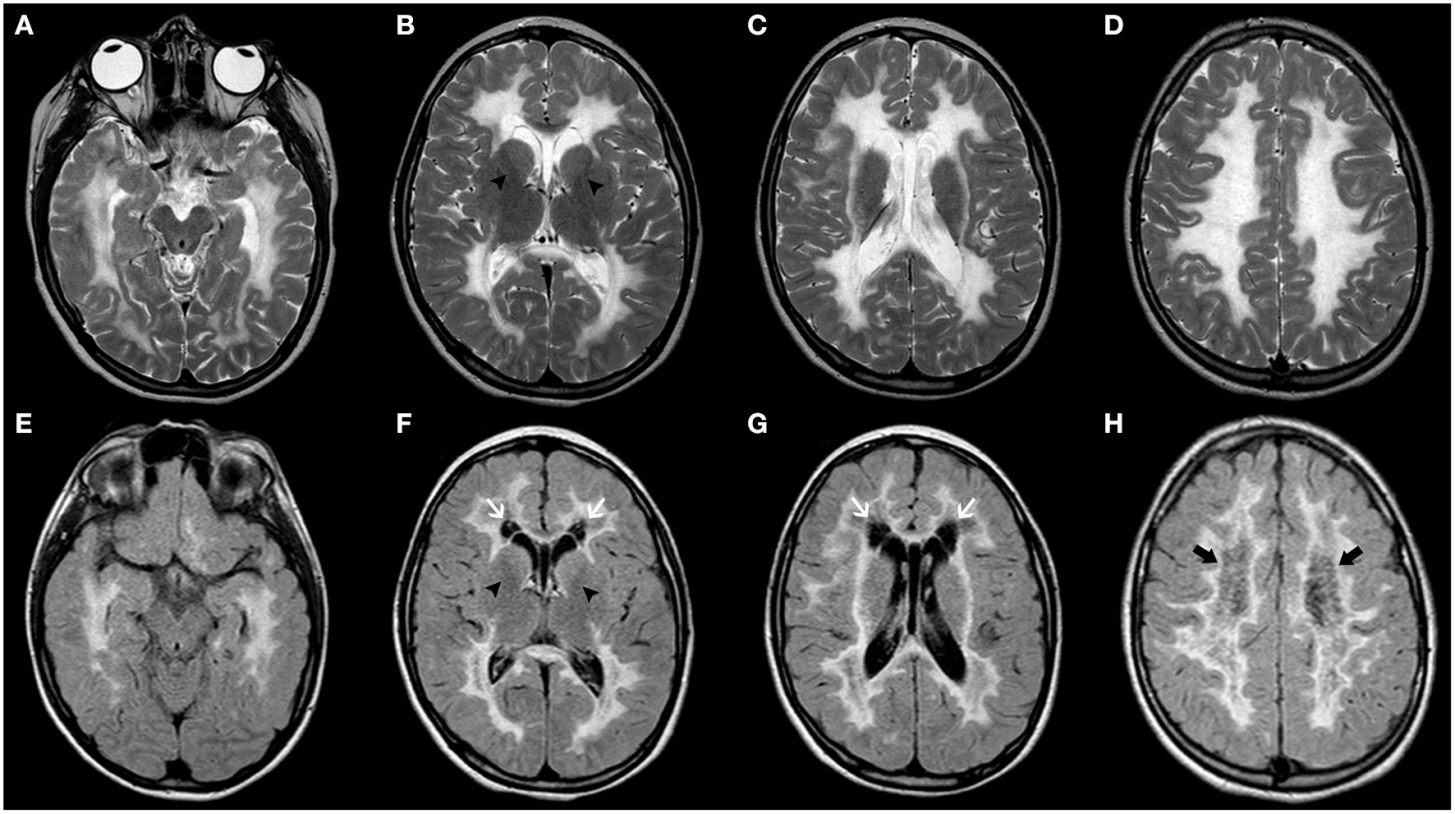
Imaging characteristics of Childhood Ataxia with Central Hypomyelination/Vanishing White Matter Disease (CACH/VWMD). Axial T2WI (A–D) and FLAIR (E–H) of an 11-year-old boy with CACH/VWMD demonstrates bilateral, confluent, symmetric periventricular, and deep white matter hyperintensity, with relative sparing of the anterior limb of the internal capsule (black arrowheads) and the subcortical white-matter/U-fibers. There are areas of cystic degeneration around the frontal horns (white arrows) and in the centrum semi-oval bilaterally (thick black arrows), with some radiation stripes depicted within the latter location.
